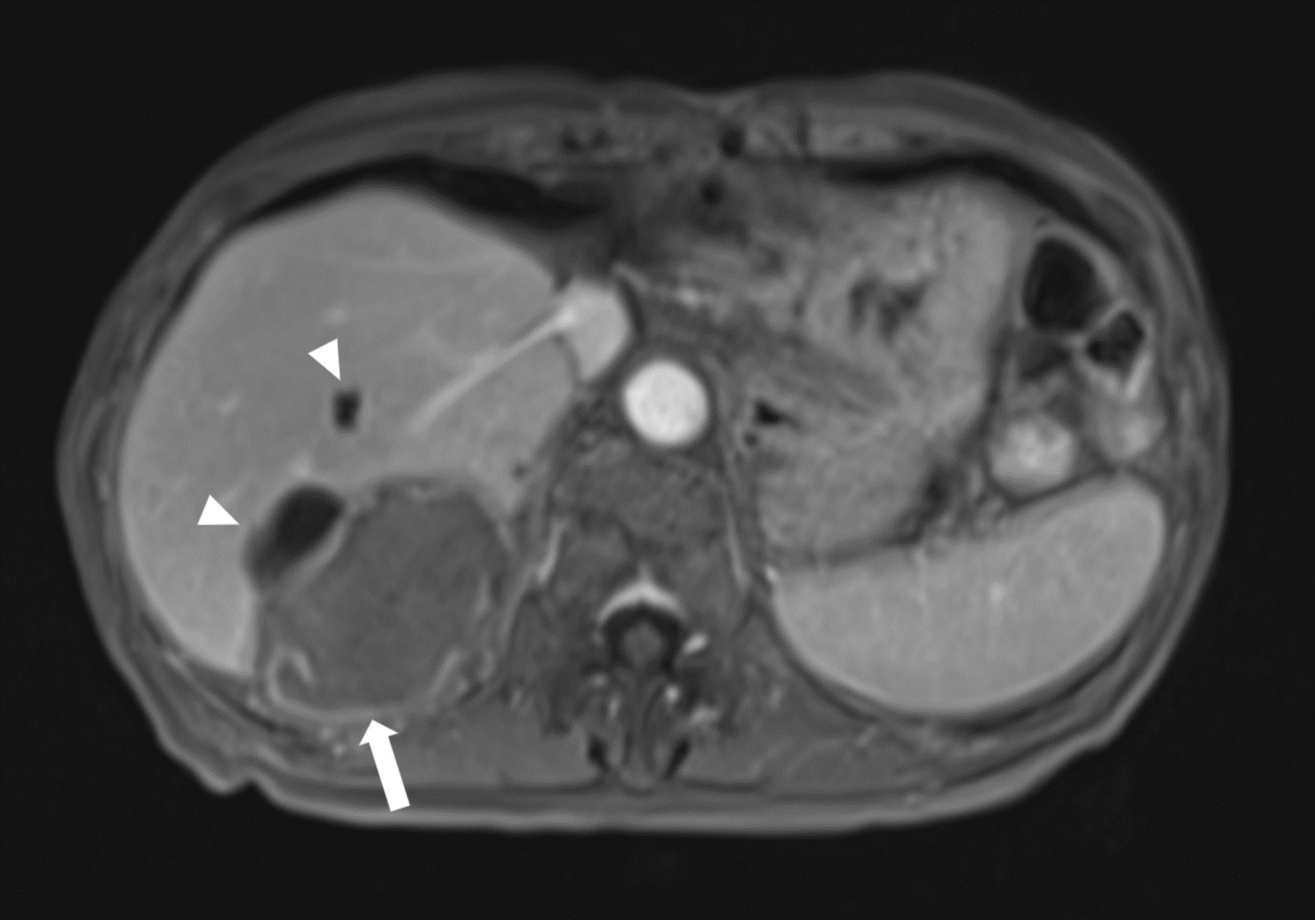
Axial MR image, T1-weighted 3D gradient-echo, late arterial phase Post-treatment ablation cavity without enhancing nodules or masses (arrow). Rim enhancement of the cavity is consistent with granulation tissue. Partially visualized anterior to the embolization cavity are two simple cysts (arrowheads). MR: magnetic resonance; 3D: three-dimensional
